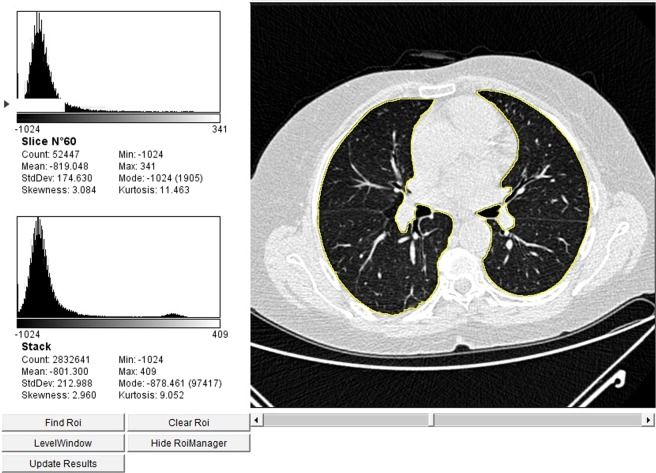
Comparison between quantitative and visual analysis of low-dose thin section volumetric lung CT in a SSc patient without visual evidence of ILD but a CII lower than the cut-off value of 0.1966 (e. g. CII = −0.3718). On the left, the figure shows the results from digital processing analysis: in the upper-left side of the image, data from the analysis of a single representative slice are given; in the lower-left side of the image the automatically generated averaged data from the analysis of all slices are given. Global pulmonary sampling has been performed and the sampled region is marked in yellow. CT = computed tomography; SSc = systemic sclerosis; ILD = interstitial lung disease; CII = computerized integrated index.
